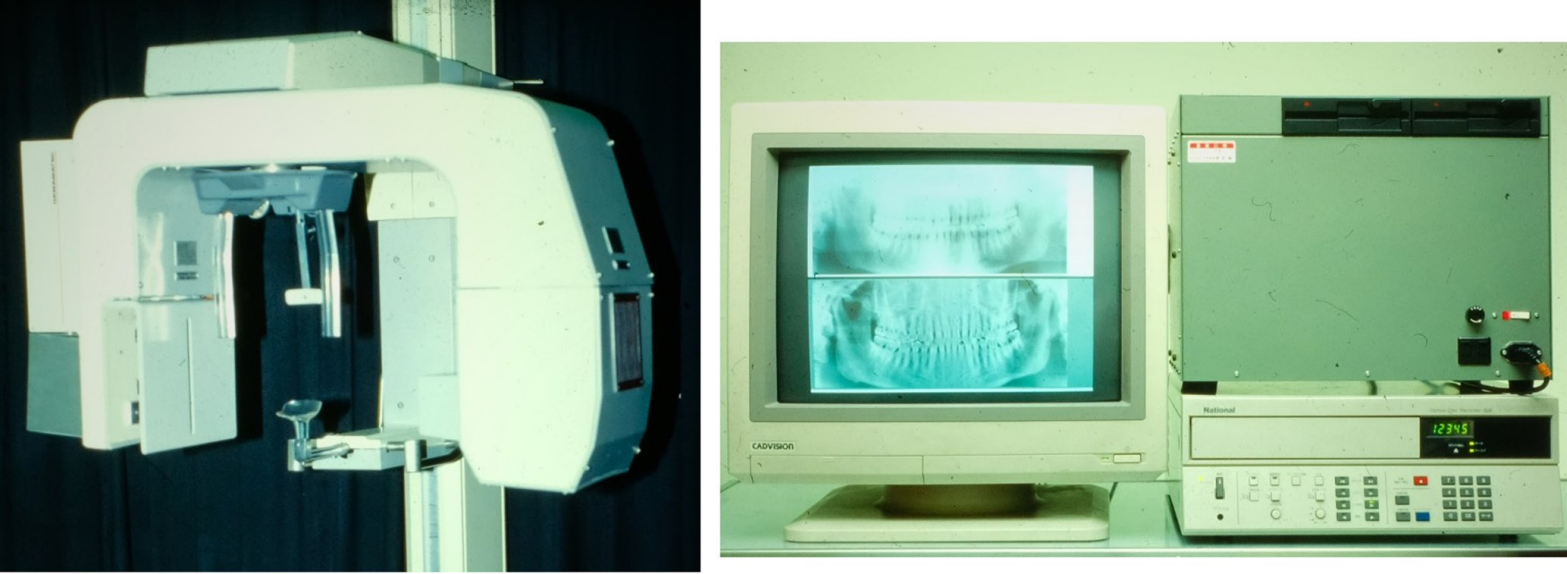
Prototype of digital panoramic tomography. This system failed because of excessive radiation doses and poor image quality. *Note*. Reprinted from “The elimination of ghost images in the development of digital rotational panoramic radiography”, by Arai Y., *Dental Radiology* 1994; 34:196, Figures 1 and 2.
